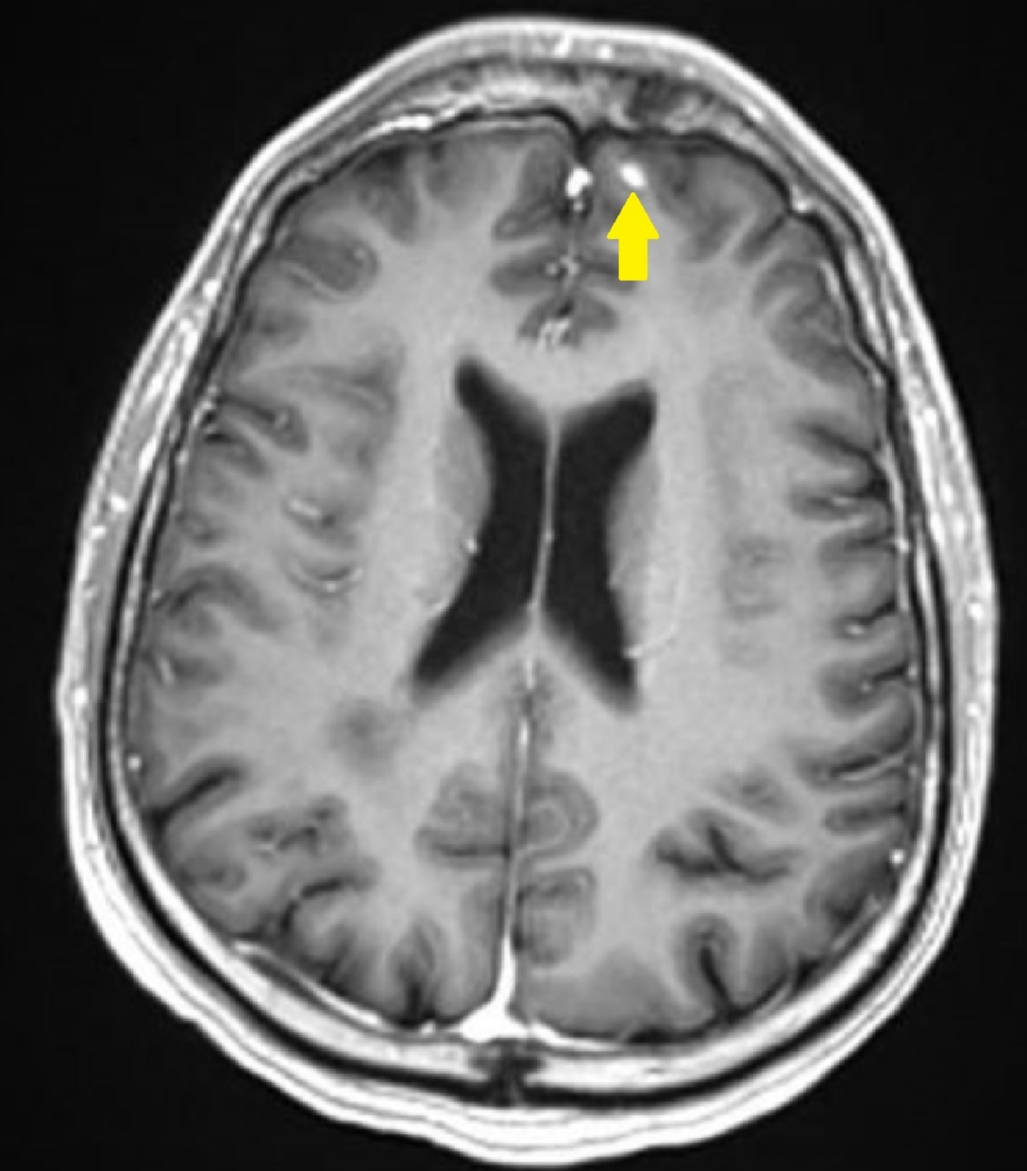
Left Frontal Lobe Metastasis Reduction Post-Treatment Transverse Brain Magnetic Resonance Imaging (MRI). Previously described 6 mm metastatic focus is again seen in the left frontal region appearing smaller.
